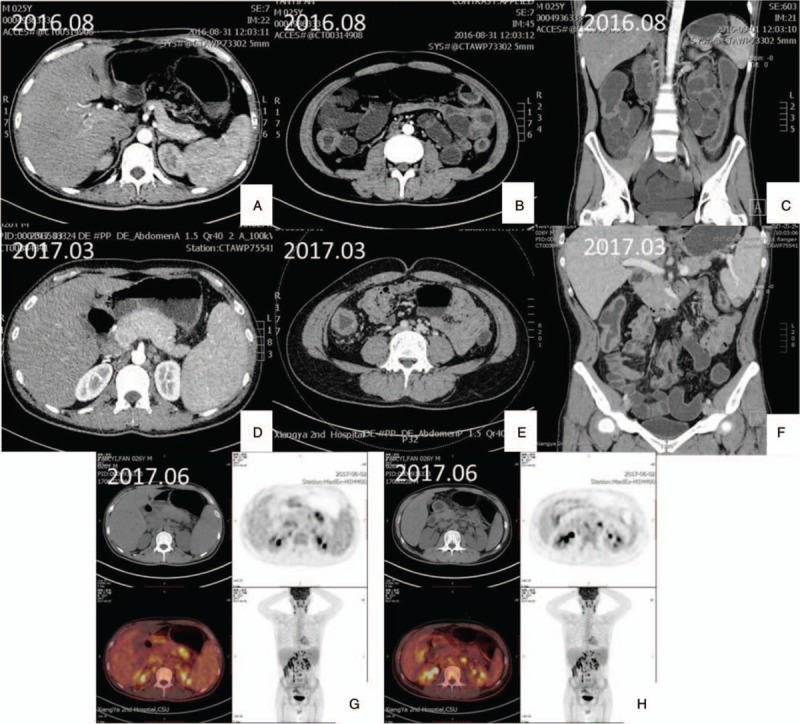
Intestine computed tomography and PET-CT. (A–C) 2016.08 An intestine computed tomography shows segmental wall of the cecum and descending colon thickened and intensive with multiple lymph nodes seen in the mesentery and retrperitoneal area. The diameter of the biggest one was 10 mm. The size of the spleen was a little bigger. (D–F) 2017.03 An intestine computed tomography shows more and bigger lymph nodes in the mesentery and retrperitoneal area with no obvious change in the colon. Liver, pancreas and spleen were bigger. There were mutiple small infarctions in spleen. (G–H) 2017.06 PET-CT shows there was a malignant tumor in the left nasopharynx wall, which may be lymphoma. Cervical and abdominal lymph nodes were swollen. Active glucose metabolism was detected in the swollen liver, pancreas and the lump in the tail of pancreas.
